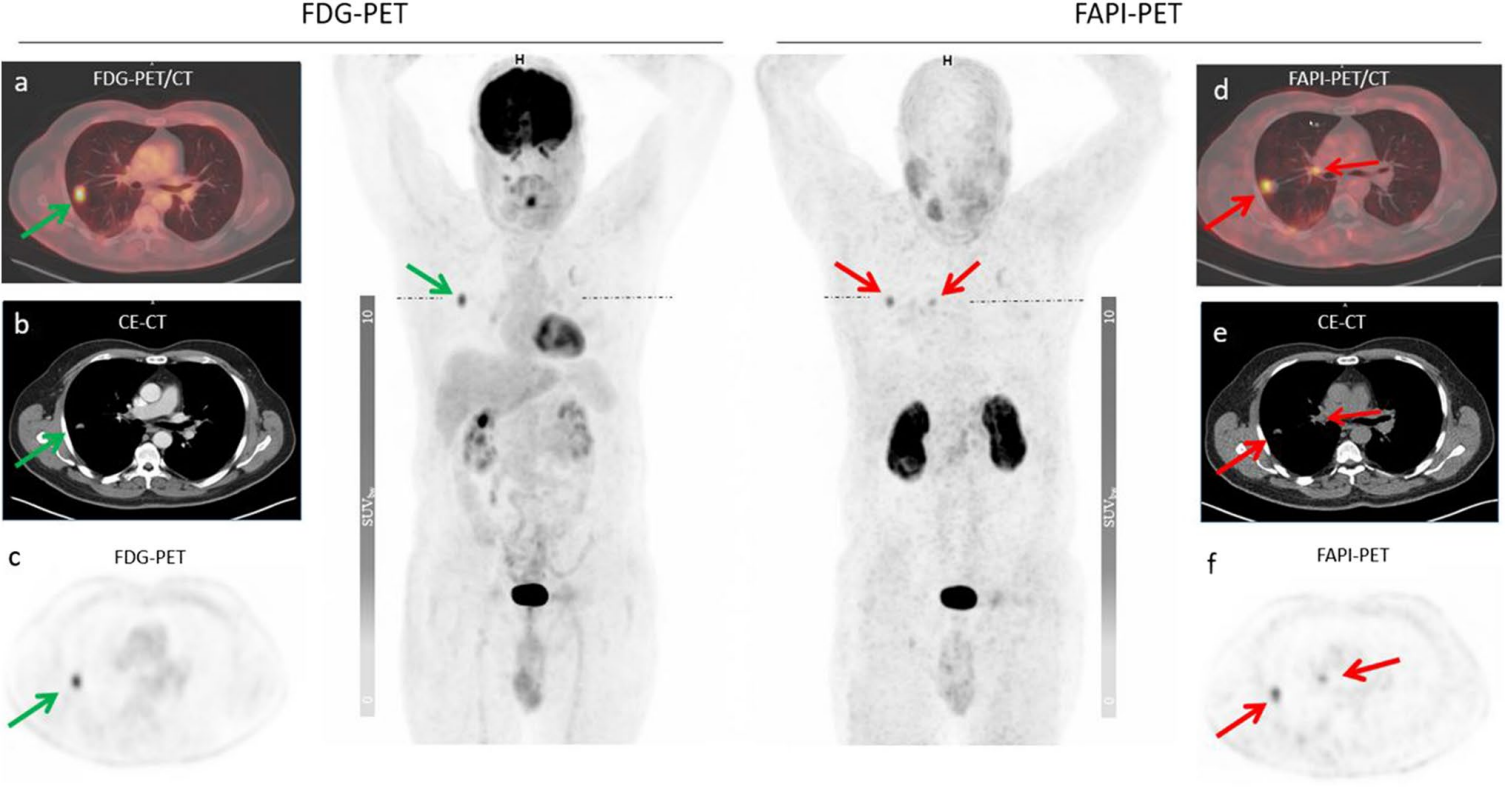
A 60-year-old patient in the 7^th^ year of follow-up for bladder cancer underwent restaging due to a suspicious lung finding on the right side. The PET/CT scan shows [^68^ Ga]FAPI avid hilar lymph node metastasis (red arrow) as well as both [^68^ Ga]FAPI and [^18^F]FDG avid pulmonary metastasis in the right lung. The quantified uptake in the hilar lymph nodes on [^68^ Ga]FAPI (d -f) was SUV_max_ 5,5 compared to the [^18^F]FDG uptake (a–c.) with an SUV_max_ 1,4 and in the lung metastasis (green arrow) on the right side on [^68^ Ga]FAPI was SUV_max_ 7,0 compared to the [^18^F]FDG uptake with an SUV_max_ 6,3 (green arrow = [^18^F]FDG, red arrow = [^68^Ga]FAPI)
